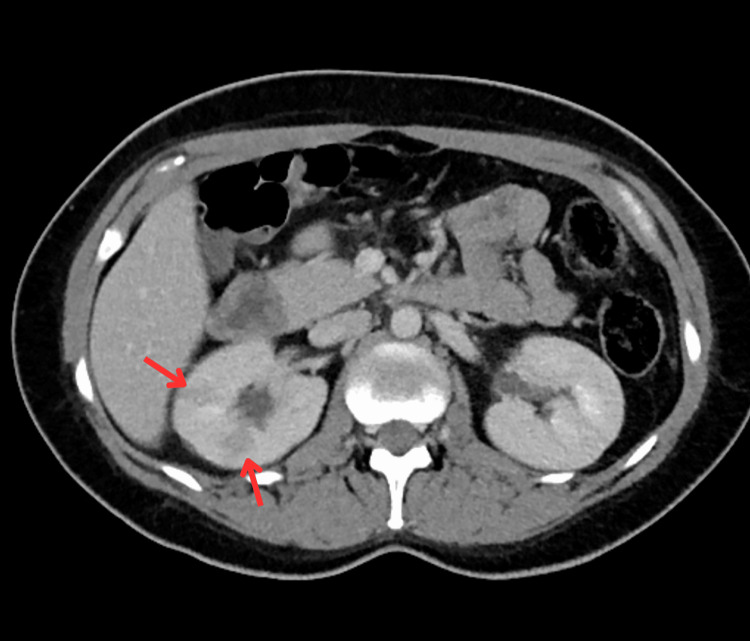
CT imaging of the mother showing hypoenhancement in the right kidney consistent with pyelonephritis
